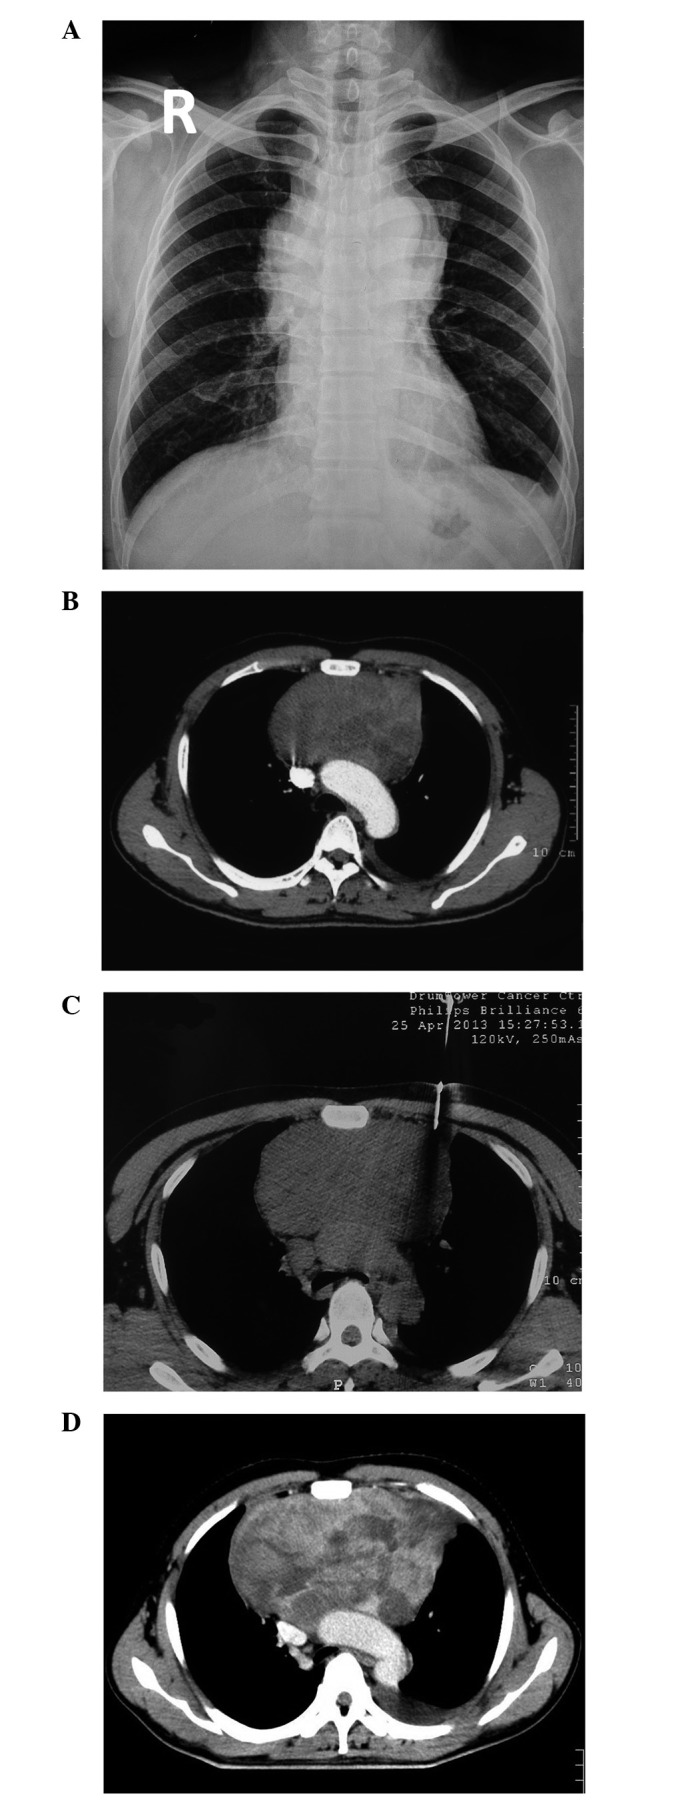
(A) Chest radiography revealied the mediastinal mass on April 23, 2013. (B) Chest computed tomography (CT) revealed a large anterior mediastinal tumor invading the great vessels on April 23, 2013. (C) A percutaneous core cutting needle biopsy of the anterior mediastinal tumor was performed under CT guidance. (D) Chest computed tomography re-examination revealed that the anterior mediastinal tumor was enlarged 1 month after the initial CT scan (May 23, 2013).
